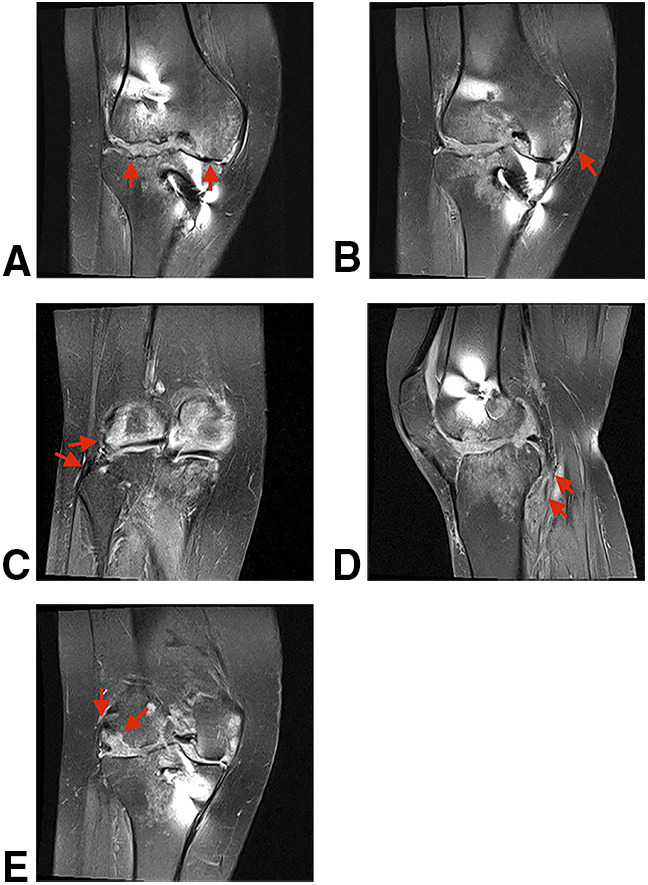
Preoperative MRI of the right knee showed extensive medial and lateral meniscus loss (A), decreased thickness of the MCL (B), LCL partial tear (C), popliteus muscle atrophy with thinning of the tendon part (D), extensive chondral loss with cortical erosions in both femoral condyles, and an occult lateral tibial plateau fracture (E).
